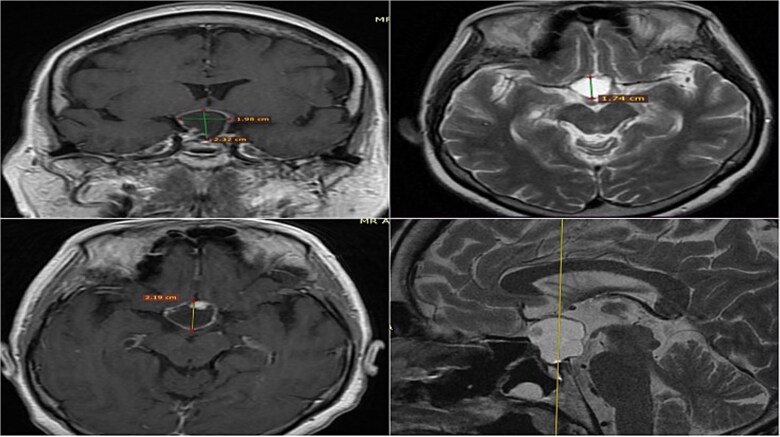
MRI of sellar region tumor in contrasted T2 and T1 sequences in axial, coronal and sagittal sections.
